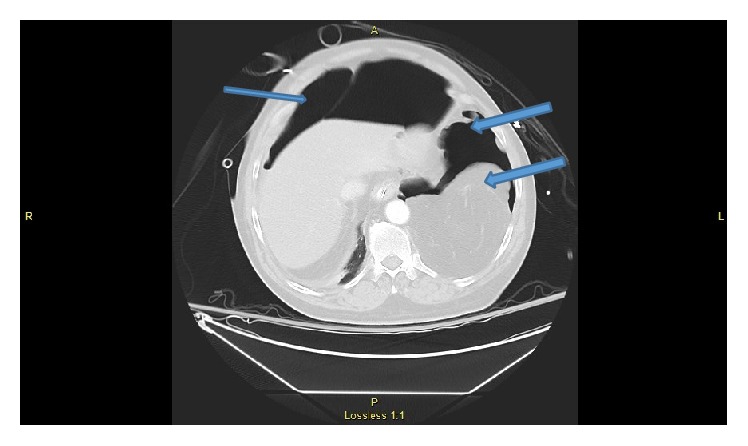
CT scan of abdomen showing pneumothorax on both sides and also herniation of omentum in left thoracic cage.
